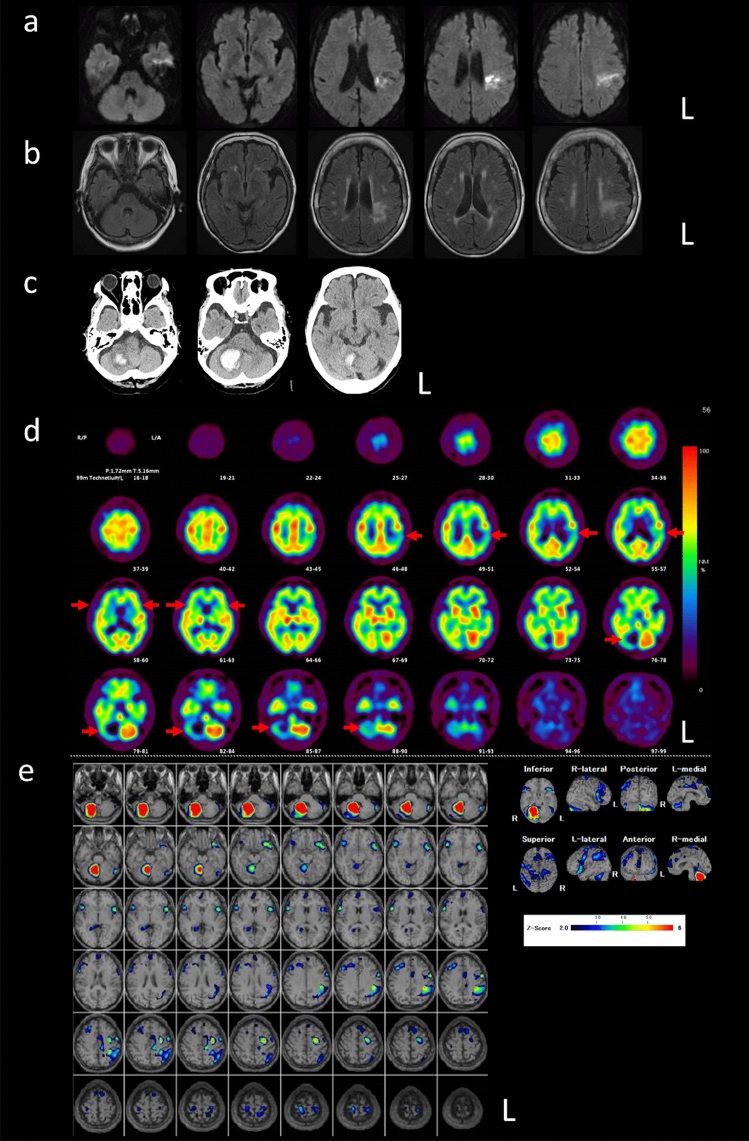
a, b Magnetic resonance imaging on the initial admission. Diffusion-weighted imaging (a) and fluid-attenuated inversion recovery imaging (b) showed a cerebral infarction extending from the left supramarginal gyrus to the anterior part of the angular gyrus. c Brain computed tomography on the second admission showed a hemorrhage in the right cerebellar hemisphere. d Single photon emission tomography (SPECT) with technetium-ethyl cysteinate dimer (^99^Tc-ECD) taken 23 days after the second admission revealed decreased cerebral blood flow (CBF) in the bilateral lateral frontal lobes, in addition to the right cerebellar hemisphere and the left inferior parietal lobule (arrows). Decreased CBF in the bilateral ACA territory was also observed. e SPECT data are analyzed with the Easy Z-score imaging system, and hypoperfusion with a Z-score > 2 is shown in color
